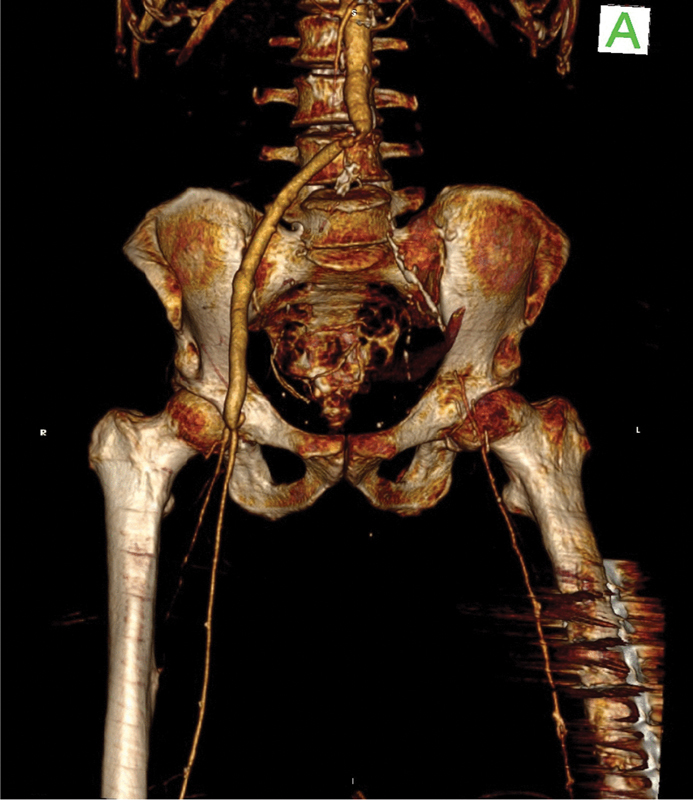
Postoperative three-dimensional computed tomography scan reconstruction. The anterior view shows the patent aortofemoral bypass and sufficient perfusion to the left leg owing to collateral circulation.
